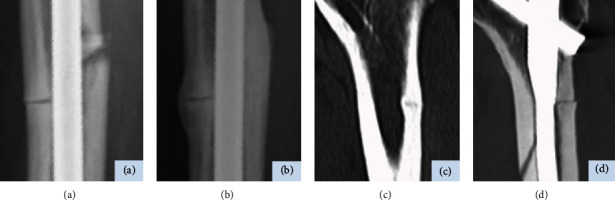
Images of the first reference case (a, b) and the second reference case (c, d). Anteroposterior radiographs showing fracture region just after surgery (a) and incomplete bone remodeling in the lateral cortex at 2 years after surgery (b). Reconstructed coronal computed tomography (CT) scans showing a thin fracture line in the lateral cortex before surgery (c) and a complete fracture at approximately 3 years after surgery (d).
